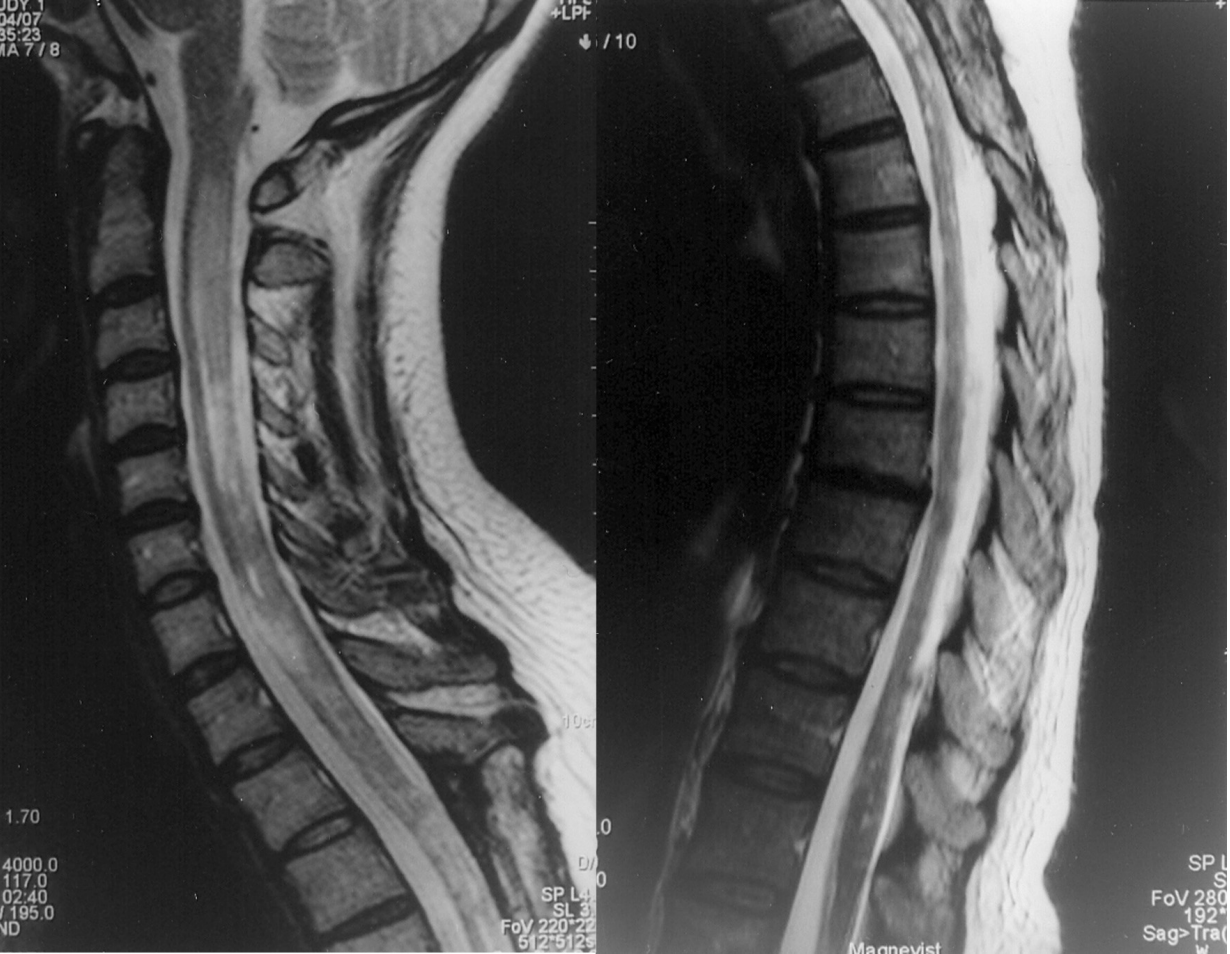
Spinal cord MRI (T2W images) performed in April 2007. MRI shows extensive T2 hyperintense changes along the spinal cord from C2 level till conus. Those changes were most prominent in the cervical and cervicothoracal spinal cord, with widening of central canal in some places and edematous enlargements, as well as with zones of necrosis.
